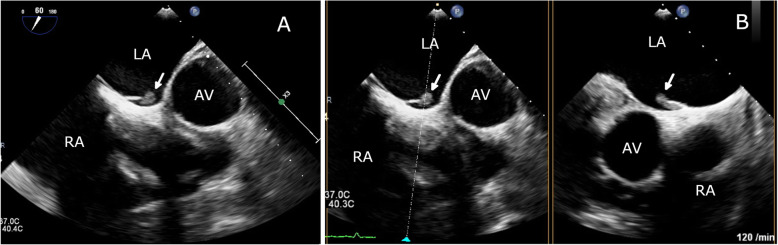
Transesophageal echocardiogram (TEE) showing LASP opening into the left atrial (LA) cavity and a 7.7 × 3 mm thrombus (arrow), with the site of attachment corresponding to the LASP (2-dimensional TEE images at 60° [a] and biplane TEE image [b] of the interatrial septum). AV, aortic valve; LA, left atrium; RA, right atrium
